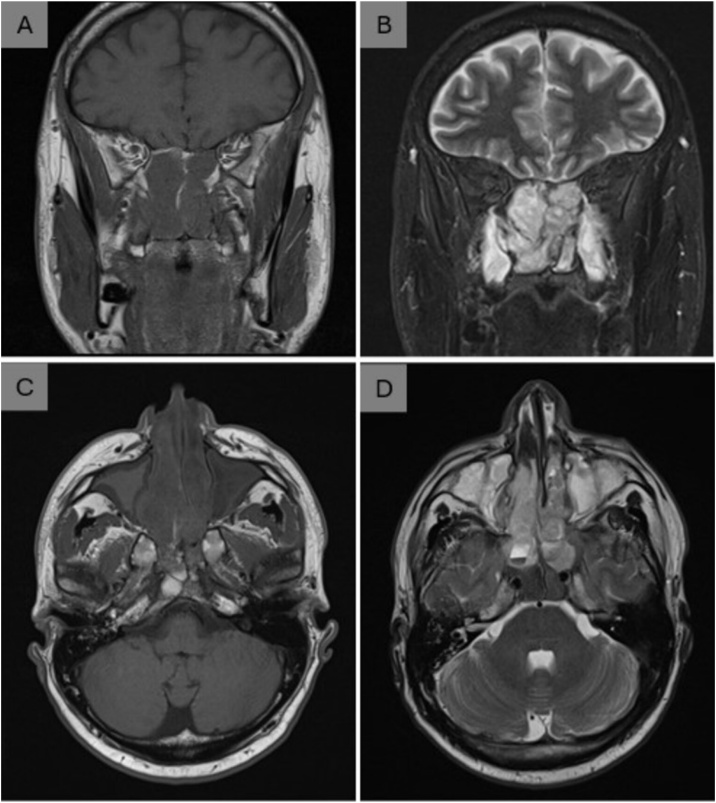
Coronal (A‒B) and axial (C‒D) MRI images showing a heterogeneous mass occupying the nasal cavities, with invasion of the ethmoid and sphenoid sinuses, skull base, and pushing the lamina papiracea.
